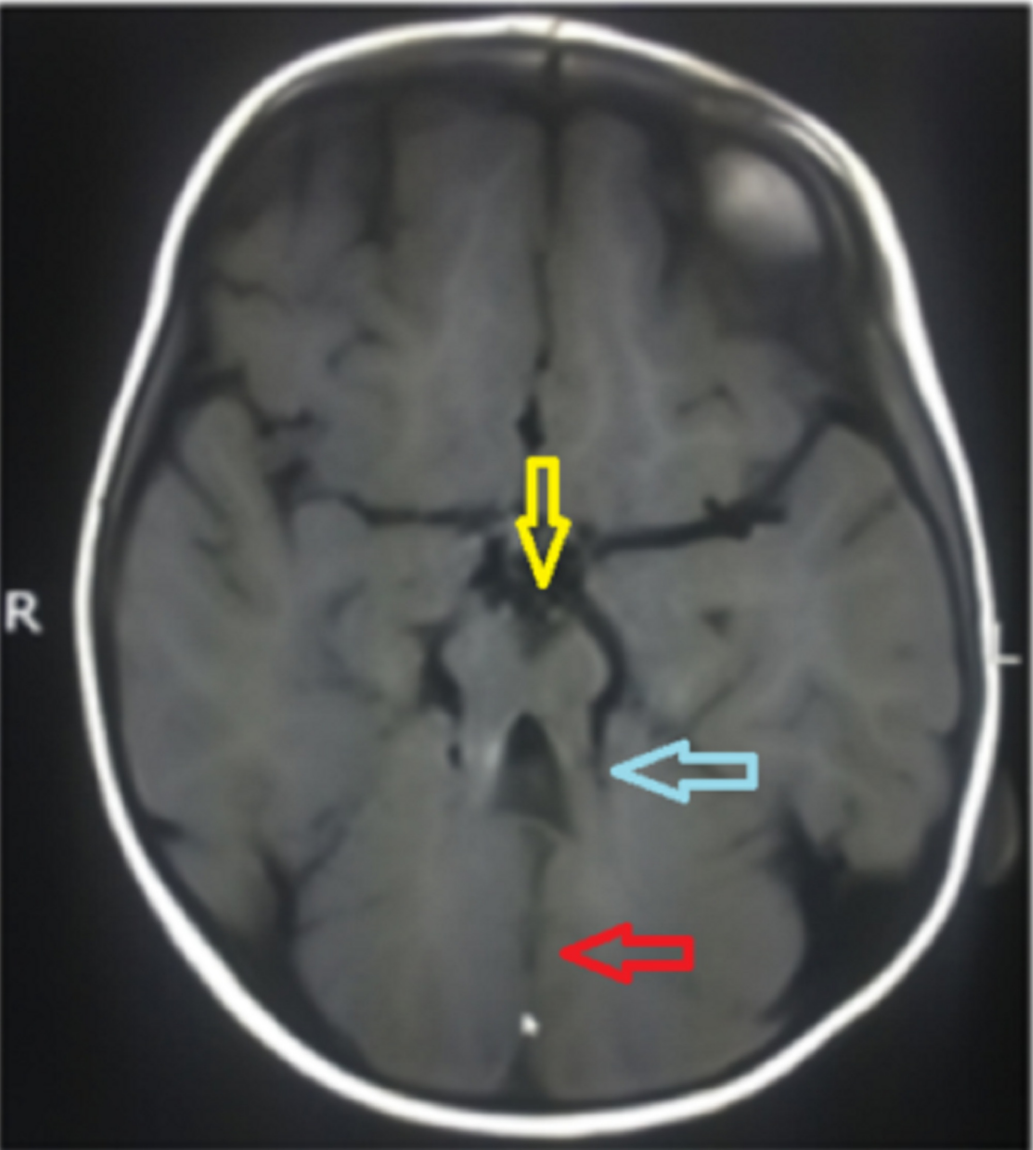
Axial T1-weighted MRI R: Right side orientation; L: Left side orientation; T1: Longitudinal relaxation time; MRI: Magnetic resonance imaging. Imaging shows deep interpeduncular fossa (yellow arrow) with thick and elongated superior cerebellar peduncles (blue arrow) imparting molar tooth appearance and aplasia of the cerebellar vermis with cerebellar hemispheres (red arrow).
